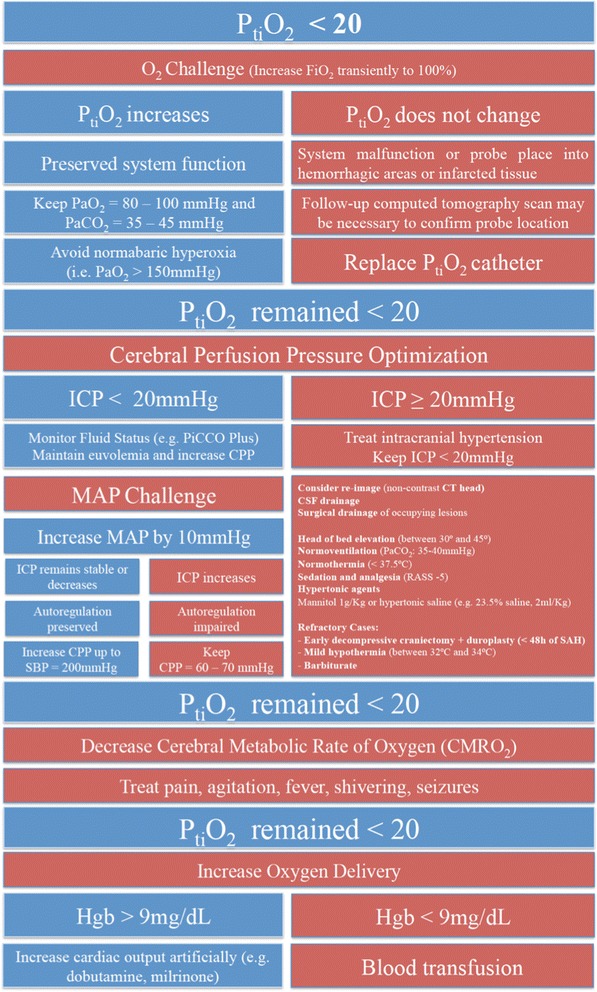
Approach to low brain tissue oxygen. Consider the combined used of P_ti_O_2_ and microdialysis catheter to detect non-hypoxic patterns of cellular dysfunction [97]. According to the manufacturer, an equilibrium time as long as 2 hours may be necessary before P_ti_O_2_ readings are stable, because of the presence of the tip surrounding microhaemorrhages. Sensor damage may also occur during insertion. Increase inspired fraction of oxygen (FiO_2_) to 100 %. If P_ti_O_2_ increases, it confirms good catheter function. Oxygen challenge to assess tissue oxygen reactivity. FiO_2_ is increased from baseline to 100 % for 5 minutes to evaluate the function and responsiveness of the brain tissue oxygen probe. A positive response happens when P_ti_O_2_ levels increase in response to higher FiO_2_. A negative response (lack of P_ti_O_2_ response to higher FiO_2_) suggests probe or system malfunction. Another possibility if there is a negative response is that the probe placement is in a contused or infarcted area. Follow-up computed tomography might be necessary in this situation to ensure appropriate probe position. Mean arterial pressure (MAP) challenge to assess cerebral autoregulation. MAP is increased by 10 mm Hg. Patients with impaired autoregulation demonstrated an elevation in ICP with increased MAP. When the autoregulation is intact, no change or a drop in ICP levels follows the elevation in blood pressure. Another way to assess cerebral autoregulation is the evaluation of the index of P_ti_O_2_ pressure reactivity. When autoregulation is intact, P_ti_O_2_ is relatively unaffected by changes in CPP, so the index of P_ti_O_2_ pressure reactivity is near zero [170]. The threshold haemoglobin (Hgb) of 9 mg/dl to indicate blood transfusion was based on a previously published P_ti_O_2_ study [171]. *CPP* cerebral perfusion pressure, *CSF* cerebrospinal fluid, *CT* computed tomography, *ICP* intracranial pressure, *PaCO*
